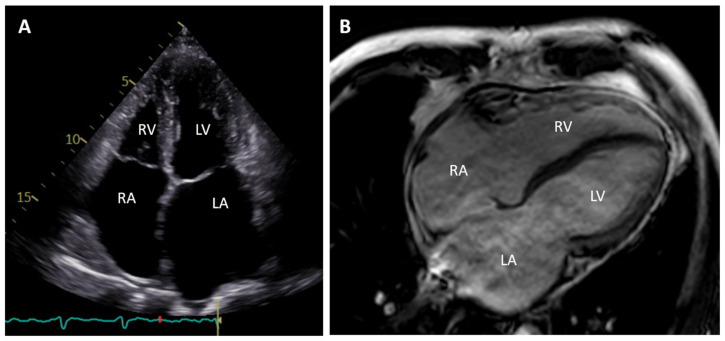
(A) Apical four chamber view during systole of an echocardiogram (B) and four chamber view of cardiac magnetic resonance image of a 50-year-old patient carrying a pathogenic *FLNC* mutation. Note the enlarged atria, normal ventricular sizes and wall thicknesses. RA = right atrium; RV = right ventricle; LA = left atrium; LV = left ventricle.
